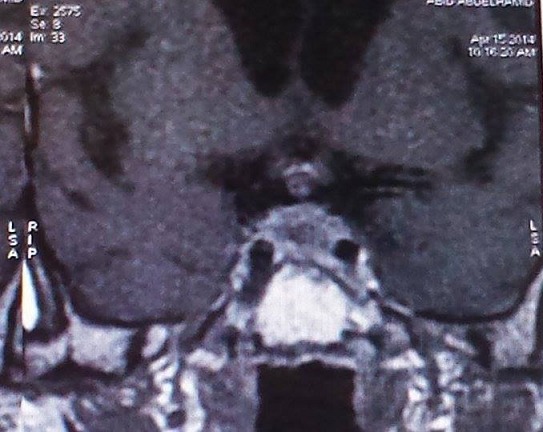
Magnetic resonance imaging: a nodular thickening of the pituitary stalk
